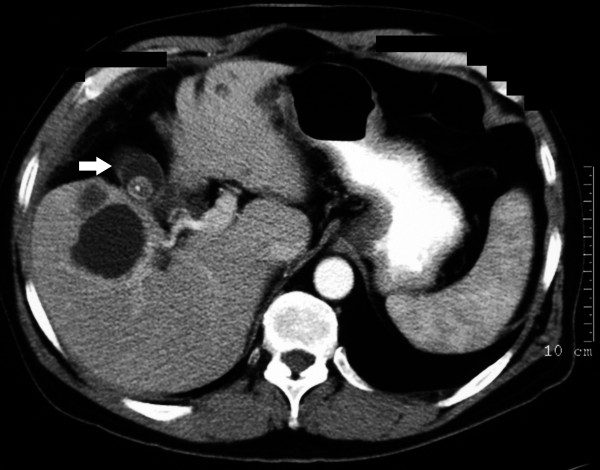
Preoperative CT scan. The scan shows a severely fibrotic, contracted gallbladder (arrow) and multiple simple liver cysts. Retrograde dissection was required in this patient (case 3) (Somatom Volume Zoom - 4 slice, Siemens AG, Erlangen, Germany).
